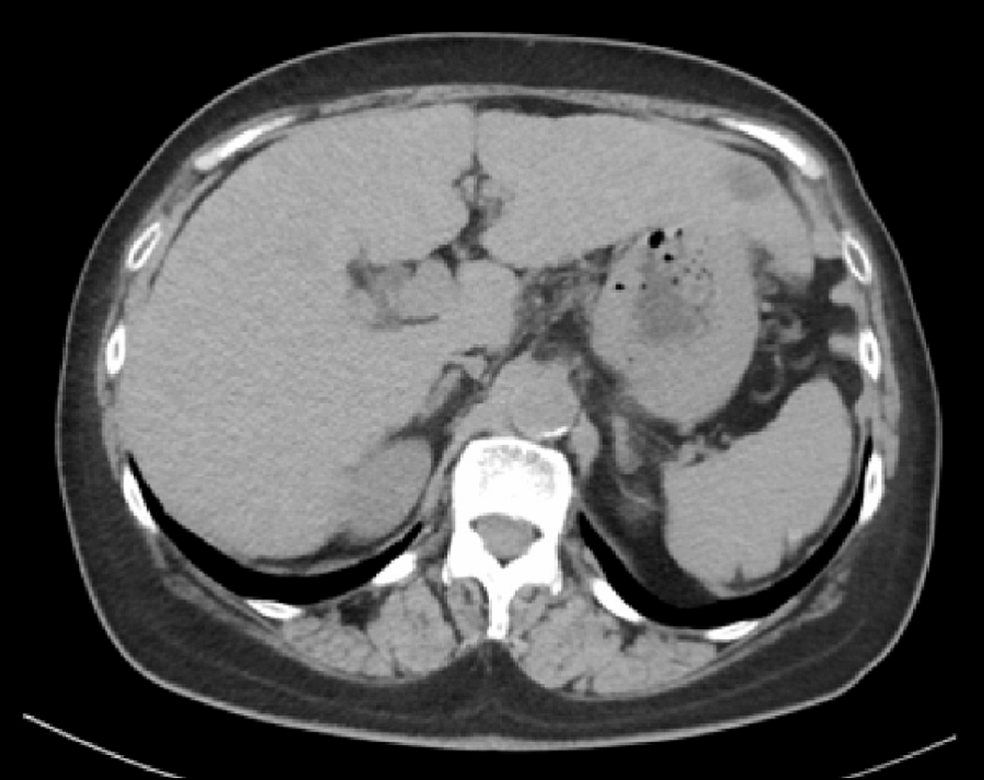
Radiological findings of the tumor in Case 2 after nivolumab administration. After nine courses of nivolumab, contrast-enhanced CT revealed an increase in the size of the target lesion and a new low-density nodule in the left lateral lobe of the liver.
